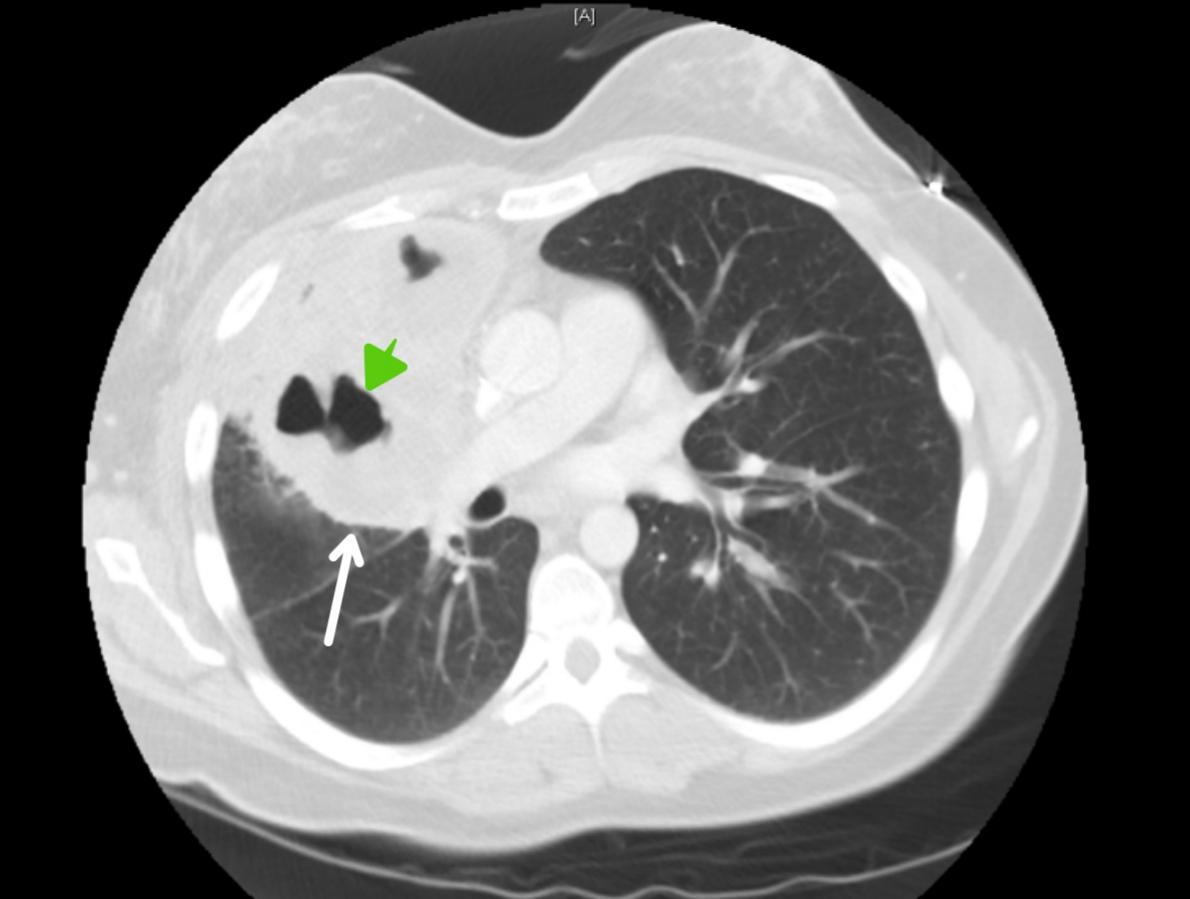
CT chest - axial view This computed tomography (CT) scan of the chest is showing a large heterogeneous mass (white arrow) with internal cavitation (green arrow) in the right middle and upper lobes.
